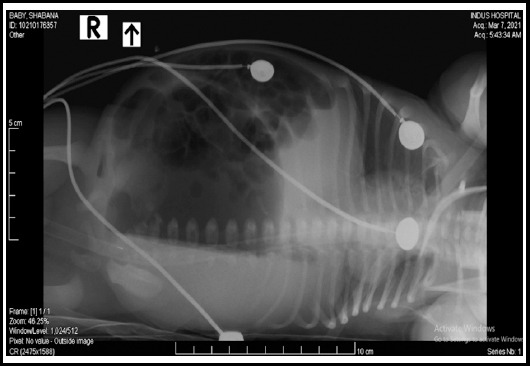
Radiograph abdomen (lateral decubitus view).
